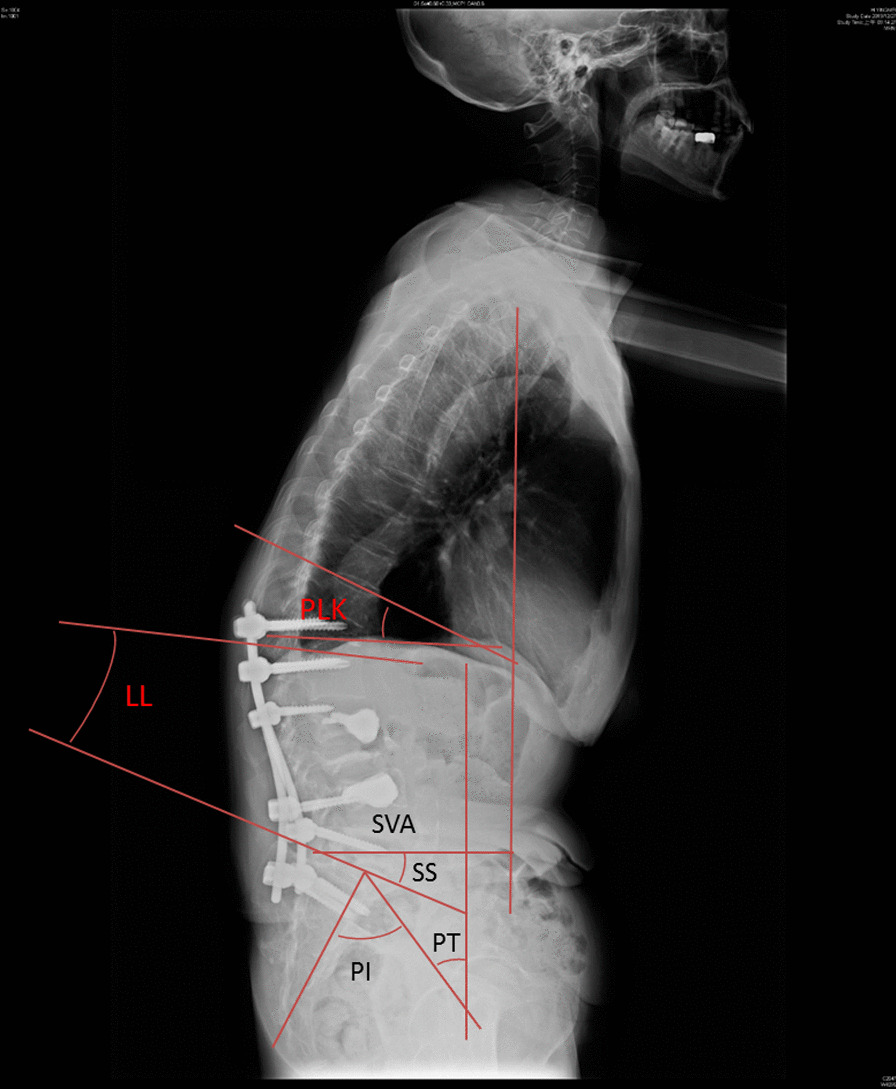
This figure demonstrated radiographic parameters on a whole spine lateral radiograph. *LL* lumbar lordosis, *PI* pelvic incidence, *PLK* proximal local kyphosis, *PT* pelvic tilt, *SS* sacral slope, *SVA* sagittal vertical axis
